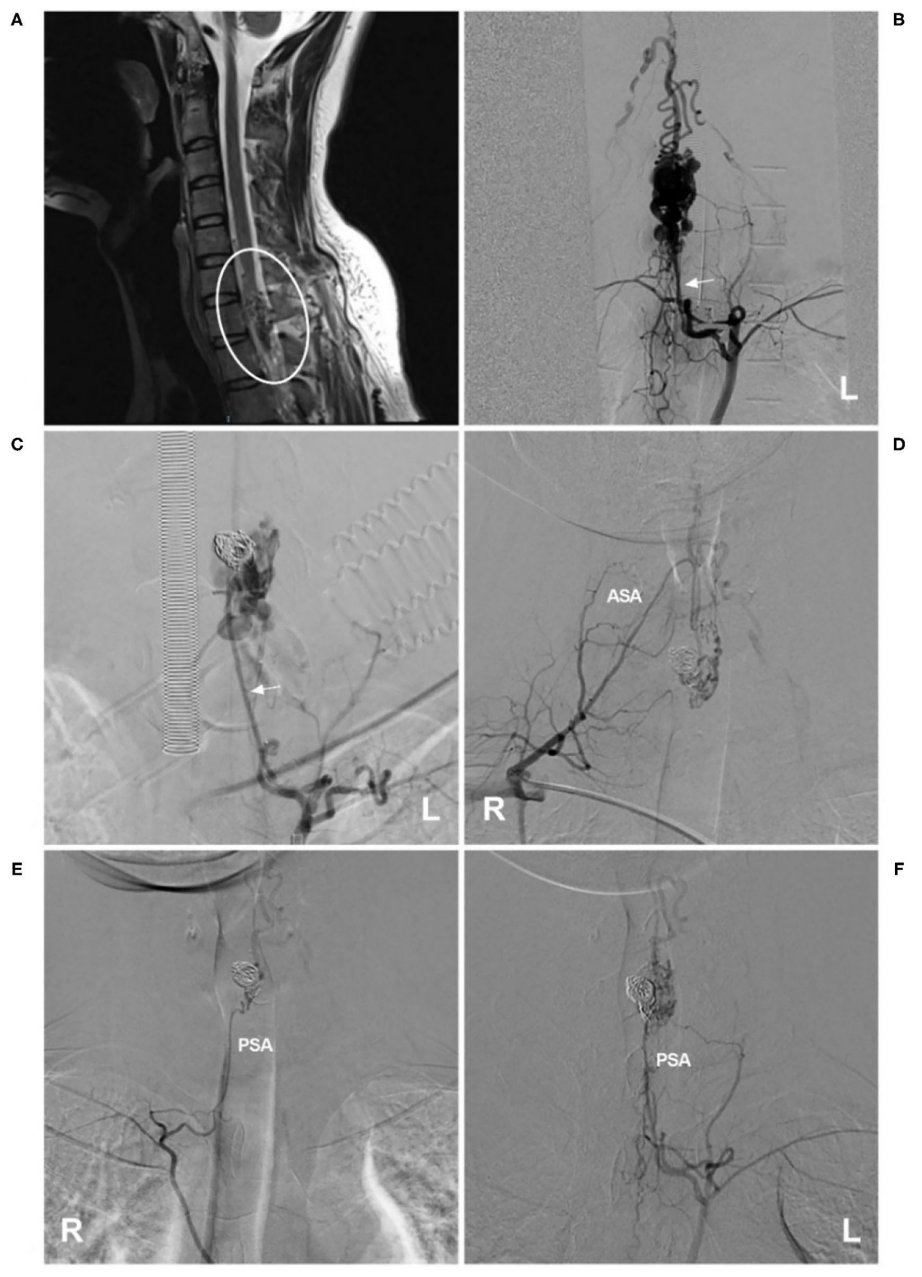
EVT of a cervical intramedullary glomus arteriovenous malformation. (A) MRI showed an intramedullary glomus AVM near the C7 level (circle). (B) Left angiogram of the supreme intercostal artery showed that the AVM was mainly supplied by the dilated radiculomeningeal artery (arrow). (C) The aneurysm in the AVM was coiled first *via* the radiculomeningeal artery (arrow), and then the NBCA was cast. (D–F) Six-month follow-up angiograms showed that the AVM had decreased. Radiotherapy was recommended. (D) shows an angiogram via the right costocervical trunk revealing that the ASA was involved as the feeder. (E,F) show angiograms via the left and right supreme intercostal arteries, revealing that the PSA was the feeder. AVM, arteriovenous malformation; ASA, anterior spinal artery; EVT, endovascular treatment; L, left; MRI, magnetic resonance imaging; NBCA, N-butyl cyanoacrylate; PSA, posterior spinal artery; R, right.
